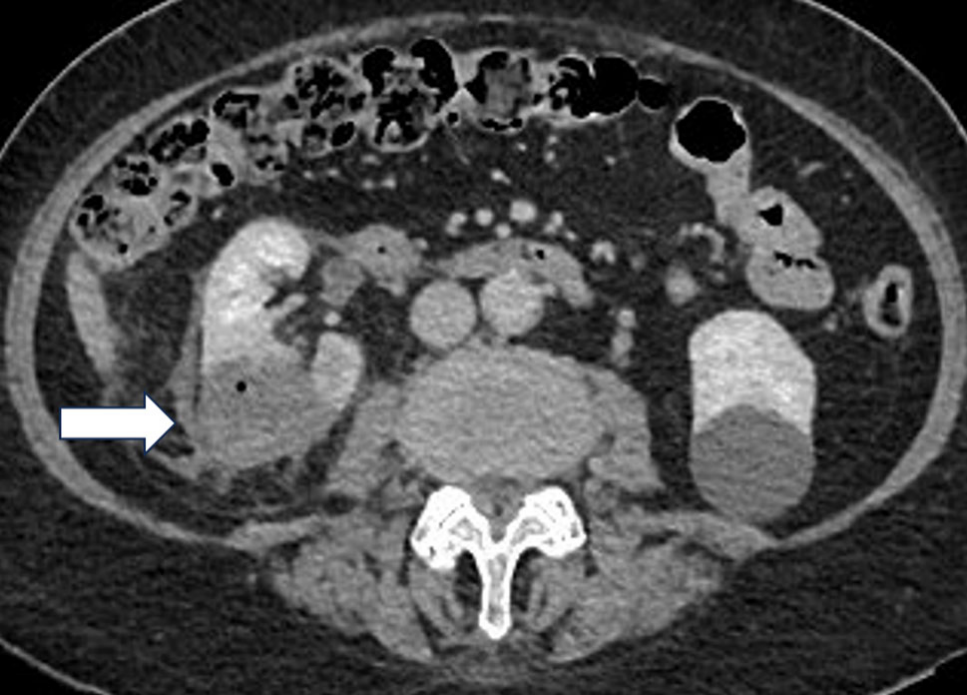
Axial contrast-enhanced CT at 36-h post-histotripsy treatment showed good treatment effect 4.27 cm (maximum diameter, core lab assessed) and completely covered the tumour. Volume outside of the planned treatment volume is due to breathing motion
